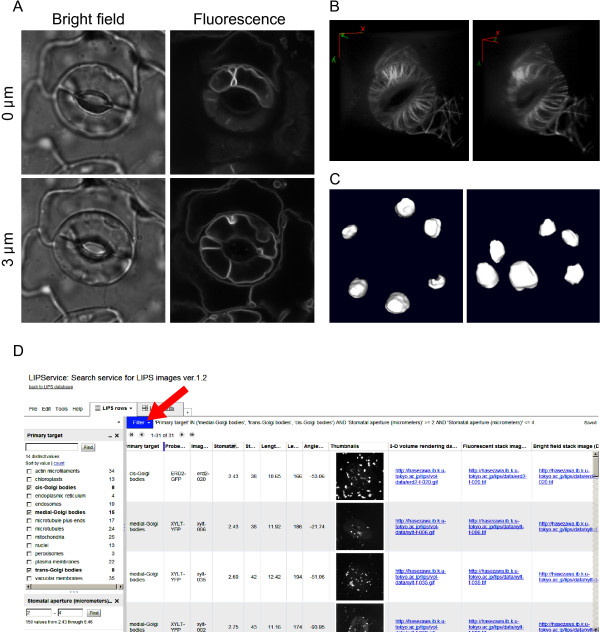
Example applications of LIPS database images. (A) Sample images from LIPS dataset I. Bright field and fluorescent images of *Arabidopsis* guard cells expressing vacuolar membrane marker GFP-VAM3 at different focal planes (0 and 3 μm). All image sizes are 500 × 500 pixels (32 × 32 μm). (B) Volume-rendering images of microtubules labeled with GFP-tubulin. The right and left images show different viewing angles. The 360°-rotation animations are available in LIPS dataset II. (C) Surface images of chloroplasts labeled with CT-GFP. The right and left images show different viewing angles. (D) Interface of the LIPService. Clicking on the “Filter” dropdown list (arrow) allows users to choose the “Filter” items (e.g., Primary target). After specifying the filter conditions, the user will see a list with a link to download image datasets I and II (in blue).
